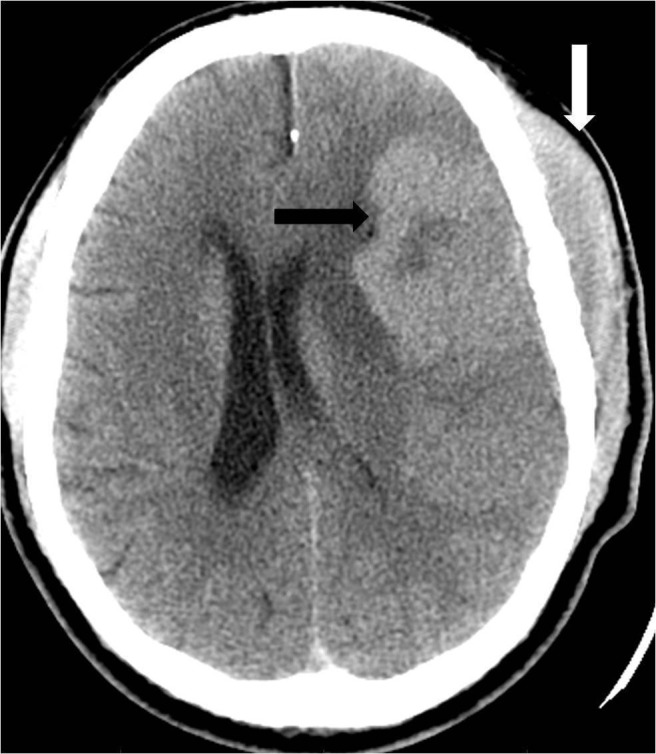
Noncontrast head computed tomography (CT) demonstrates a transcalvarial hyperdense mass with both intracranial (black arrow) and extracranial (white arrow) components.
